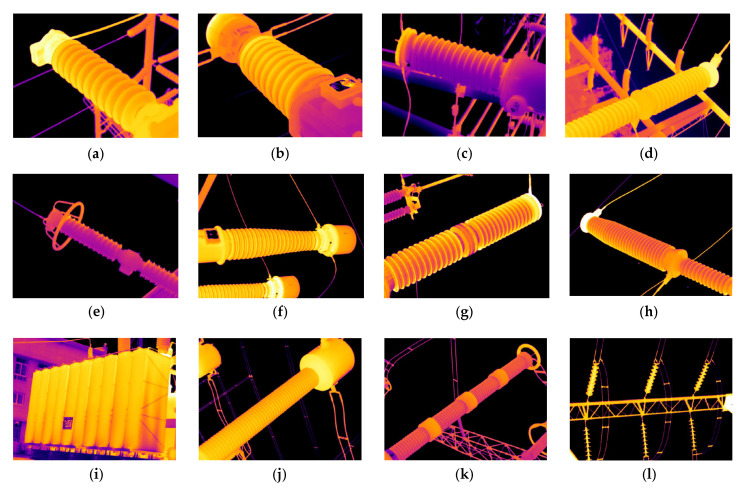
Original data and equipment categories: (a) 110 kV arrester; (b) 110 kV CT; (c) 110 kV PT (d) 110 kV breaker; (e) 220 kV arrester; (f) 220 kV CT; (g) 220 kV PT; (h) 220 kV breaker; (i) transformer; (j) 500 kV CT; (k) 500 kV PT; (l) insulator string.
